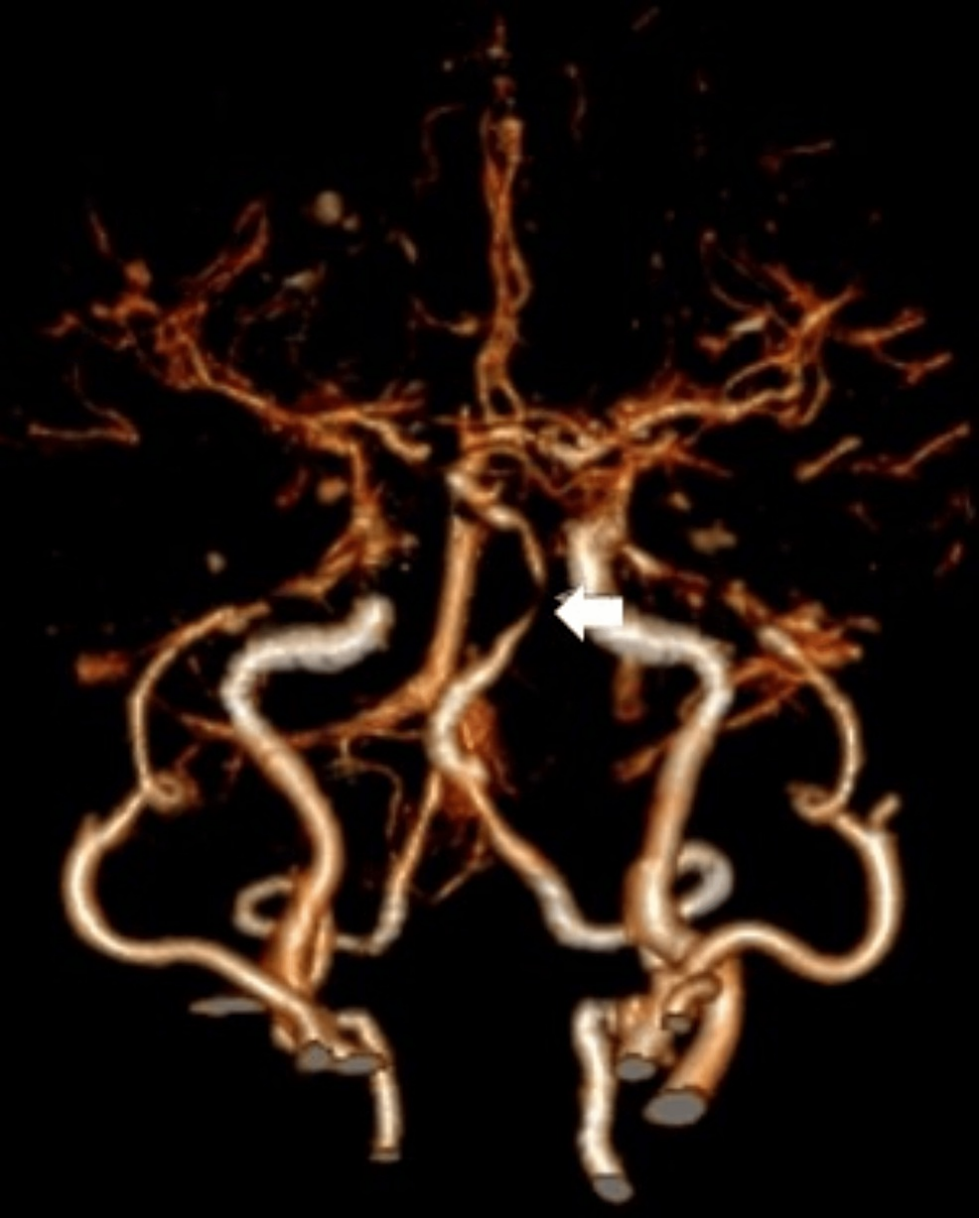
Cerebral CTA (volume rendering technique using maximal intensity projection) showed severe stenosis at the middle part of the basilar artery (white arrow). CTA: computed tomography angiography.
